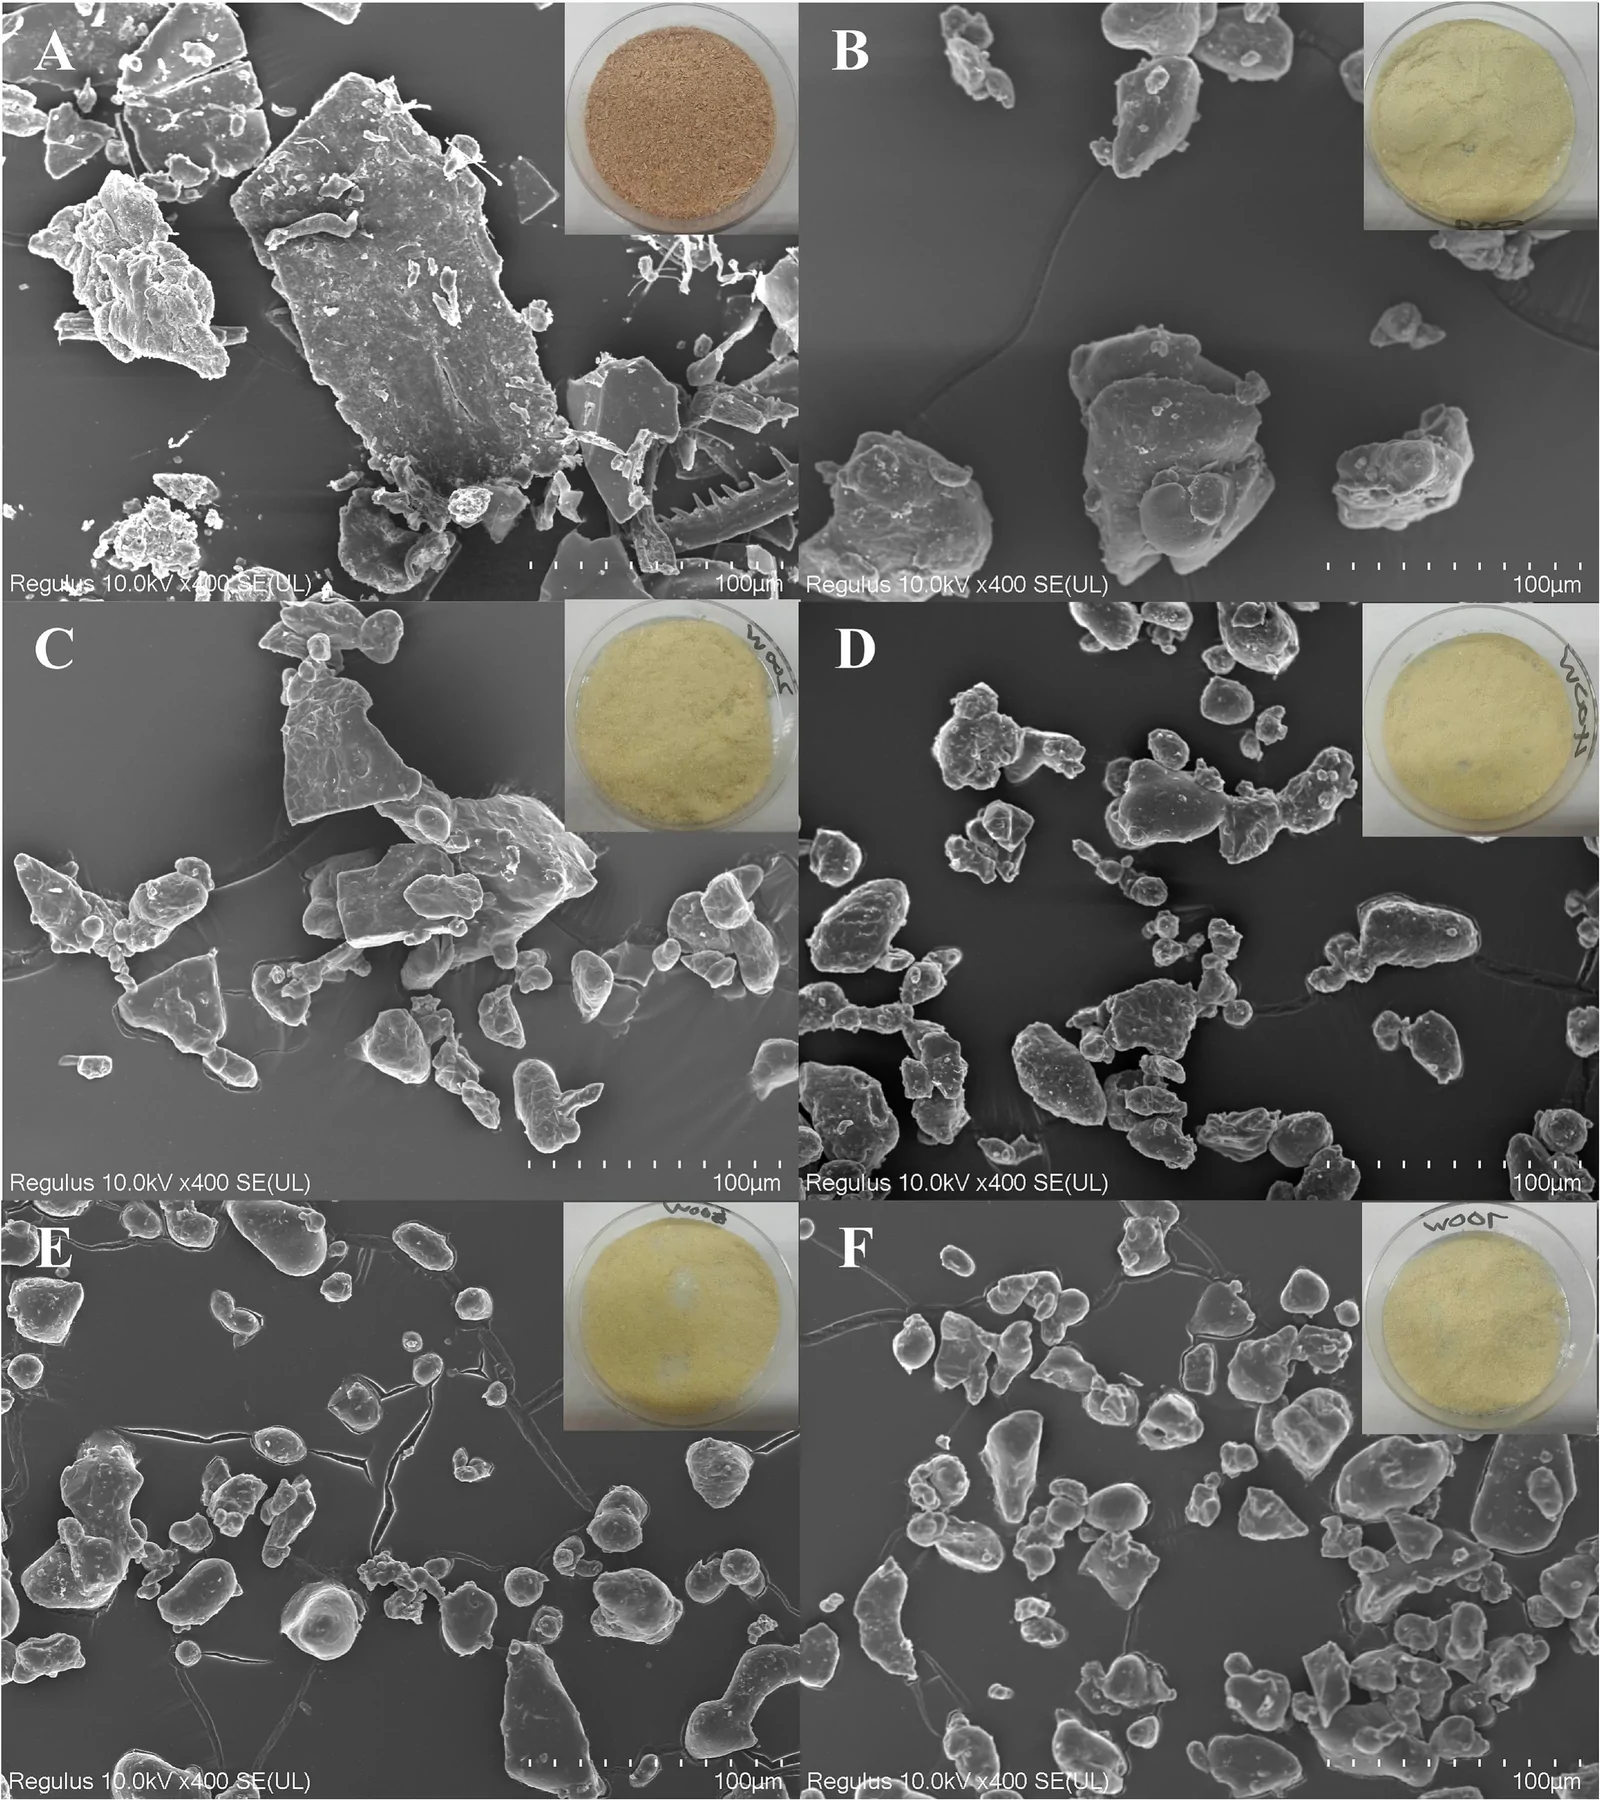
Micromorphologies (A1-F1) and appearances (A2-F2) of AKPs prepared through HUF with different ultrasonic powers. A, defatted Antarctic krill; B-F, AKP prepared through HUF. The ultrasonic powers of HUF: B, 0 W; C, 200 W; D, 400 W; E, 600 W; F, 700 W.
